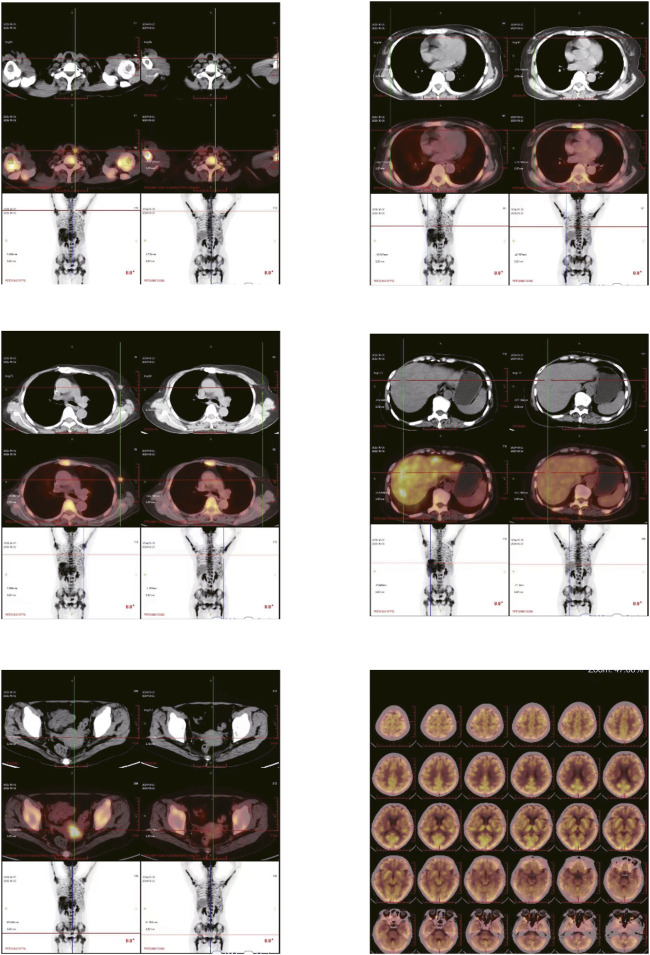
Comparison of pre- and post-treatment PET-CT findings. Baseline PET-CT showed diffuse abnormal bone marrow metabolism with osteoblastic changes consistent with bone marrow metastasis. PET-CT re-evaluation on 9 October 2025 showed enlargement of the right breast lesion, a new left breast lesion, and new liver metastases, indicating disease progression.
